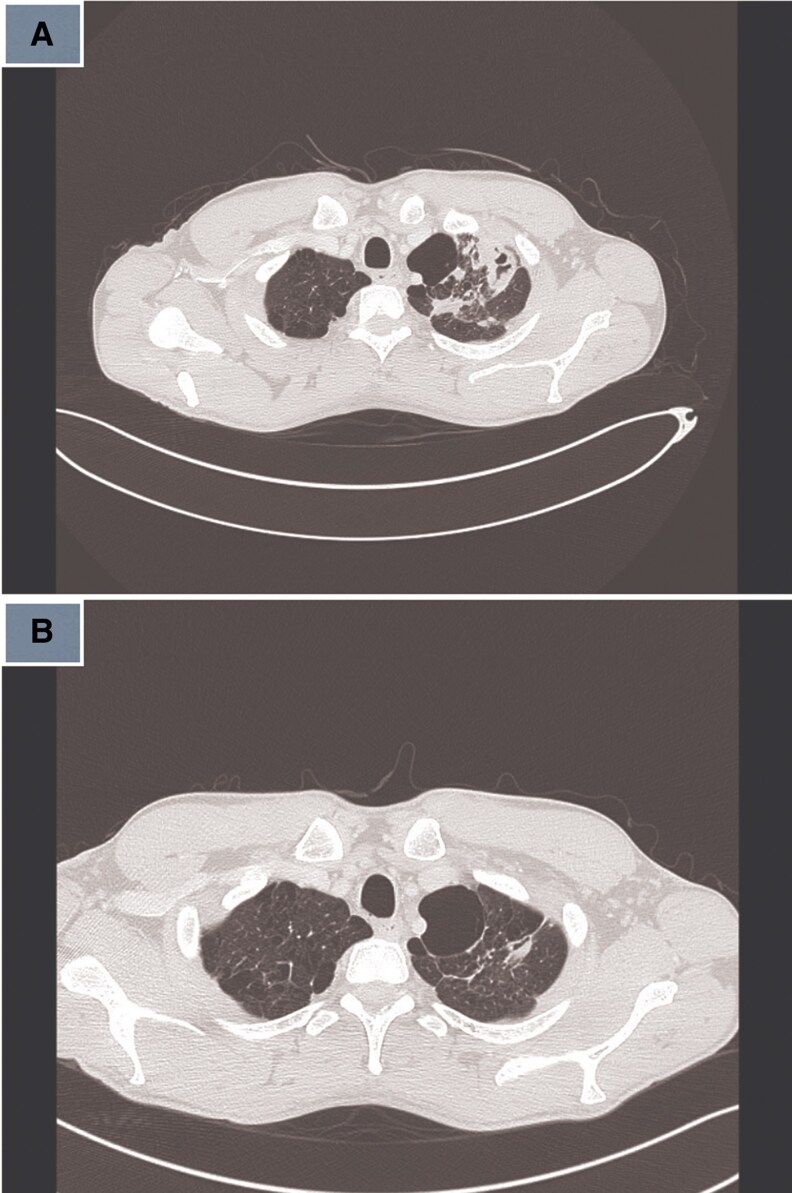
(*A*) Radiological image (eg, CT scan) showing consolidation and cavitation in a pulmonary *M riyadhense* infection (case 4; Table 2). (*B*) Follow-up imaging showing resolution of lesions posttreatment (case 4; Table 2).
